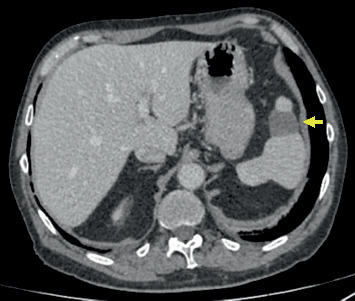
Computed tomography (CT) scan of the abdomen showing a splenic infarction. The yellow arrow indicates the infarcted area in the anterior region of the spleen.
